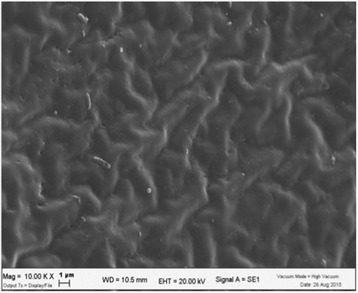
Aligner cleaned by method 9 shows clear signs of water absorption and ultrasonic cavitation
